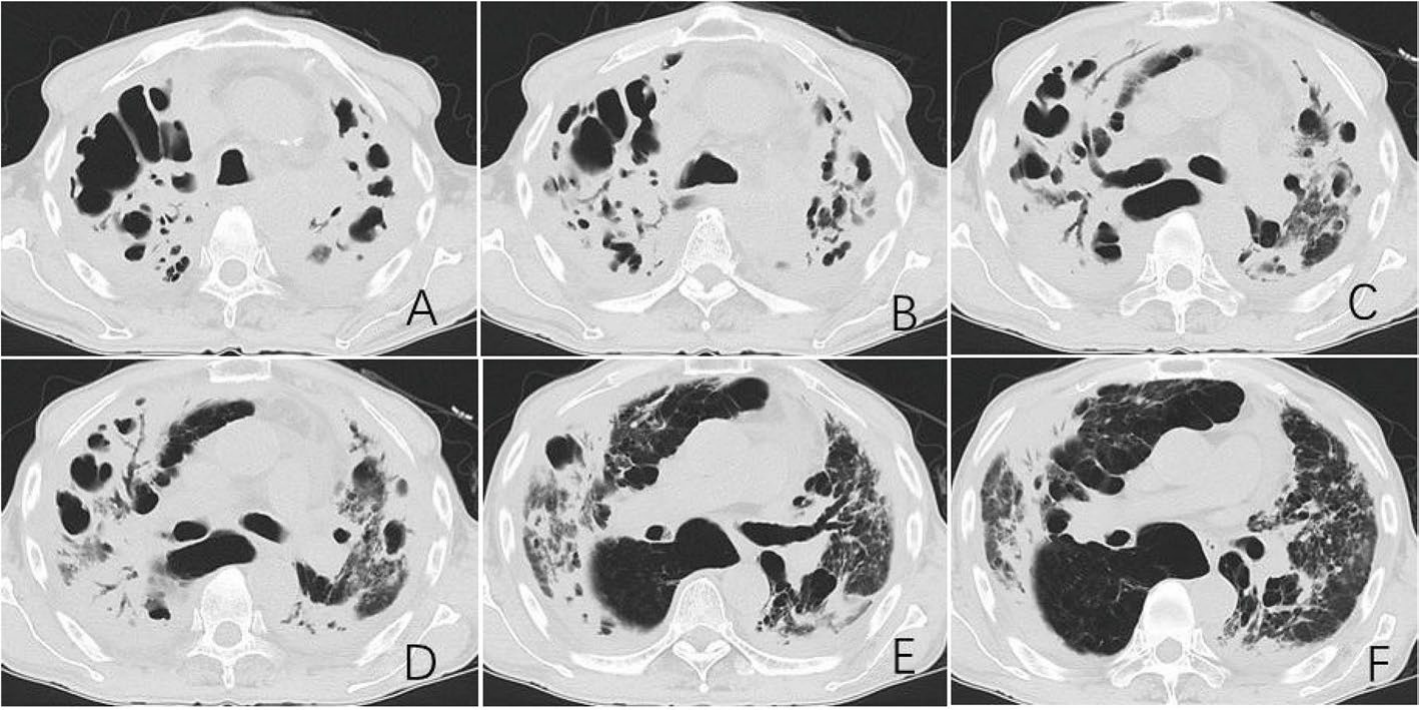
Representative chest CT findings in a 71-year-old male with DR-TB. Key features include: (i) bilateral lobar consolidations with air bronchograms; (ii) worm-eaten cavities and multiple cavities of varying sizes; and (iii) emphysema
